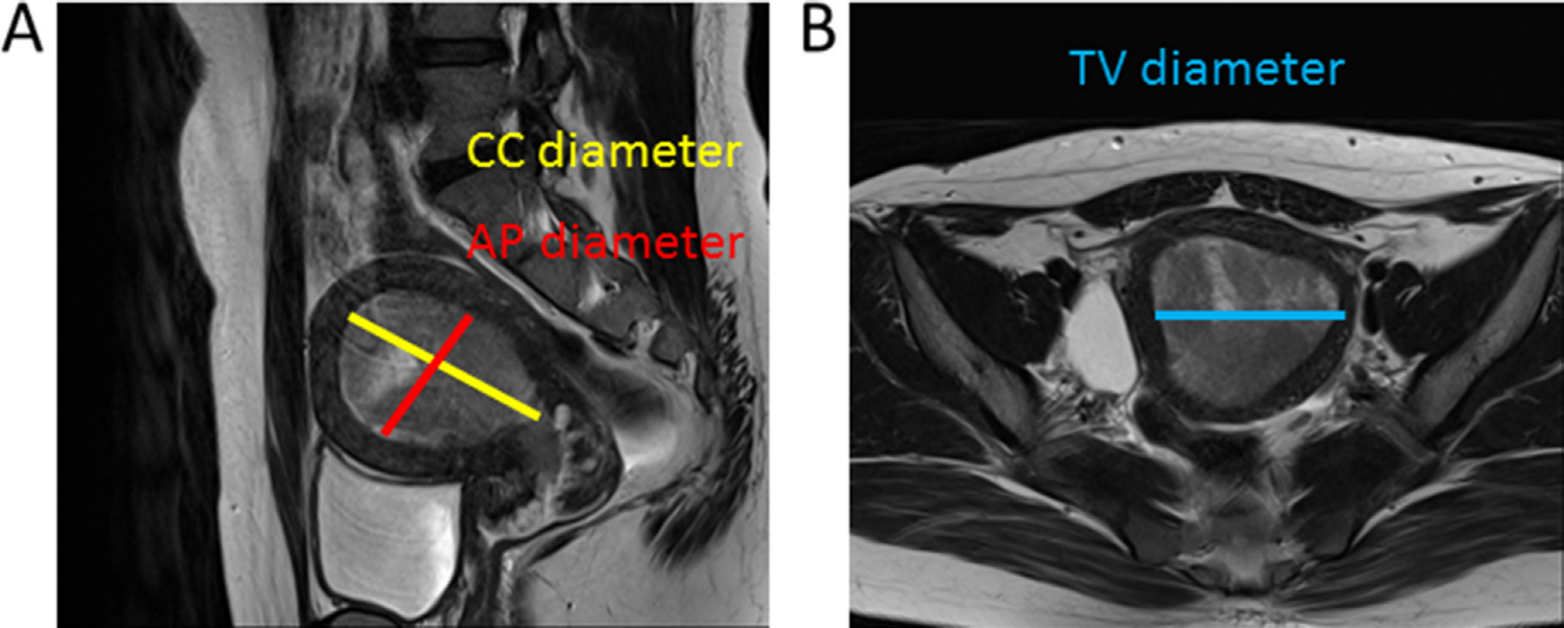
Images used to determine tumor diameter The maximum tumor diameters were determined along three orthogonal planes. AP and maximum CC diameter were determined using the sagittal oblique T2-weighted image (A), and the TV diameter was determined using the coronal image (B).
